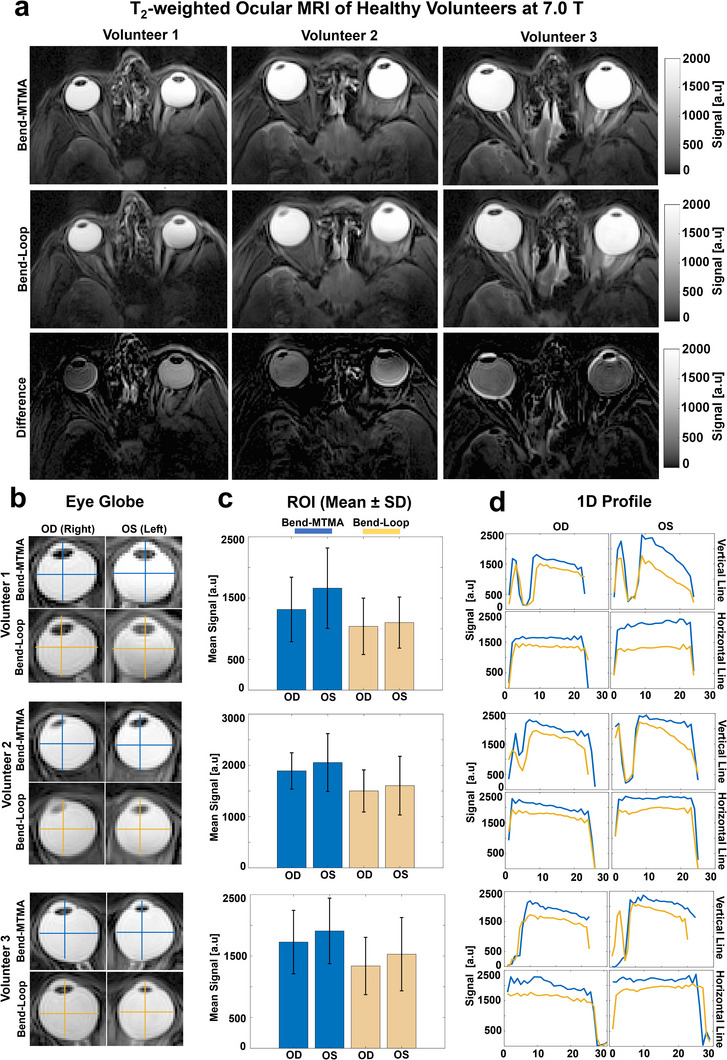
In vivo T_2_‐weighted Turbo Spin Echo (TSE) MRI in the same three healthy volunteers using the Bend‐MTMA and Bend‐Loop. (a) Axial slice images reveal increased intraocular signal for the Bend‐MTMA configuration, while the corresponding difference maps of (MTMA‐Loop) highlight the MTM‐facilitated signal enhancement. (b) Zoomed views of the eye used for eye‐globe ROI analysis. Zoomed view used the same scale as the full view above. Vertical and horizontal lines were used to extract the 1D signal profile. (c) Bar plots showing ocular ROIs’ Mean ± SD signal intensity, demonstrating consistent signal enhancement with the Bend‐MTMA over the Bend‐Loop. (d) 1D signal intensity profiles across both eyes reveal enhanced depth penetration of the signal with the Bend‐MTMA.
